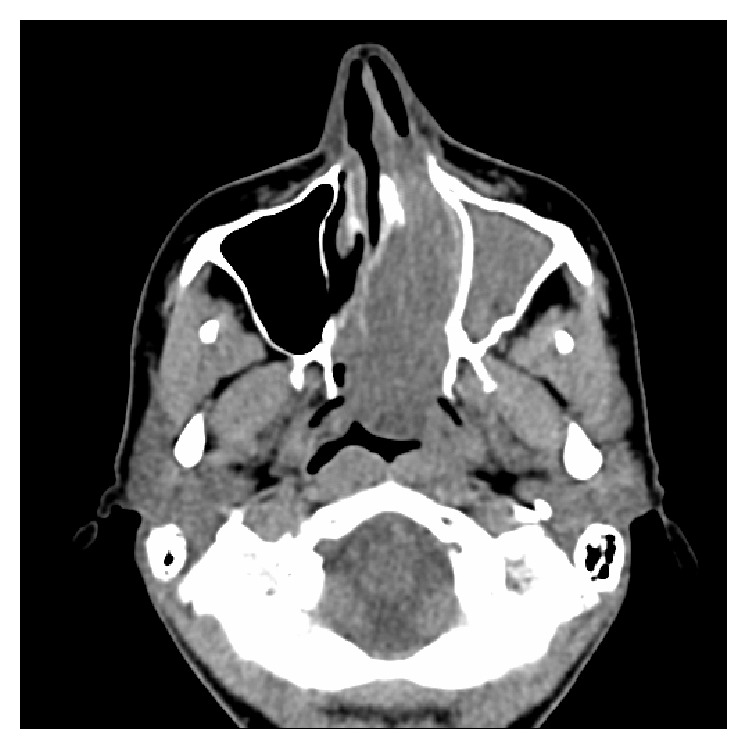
CT (axial view) showing complete opacification of left frontal, ethmoid, maxillary, and sphenoid sinuses and nasal cavity.
